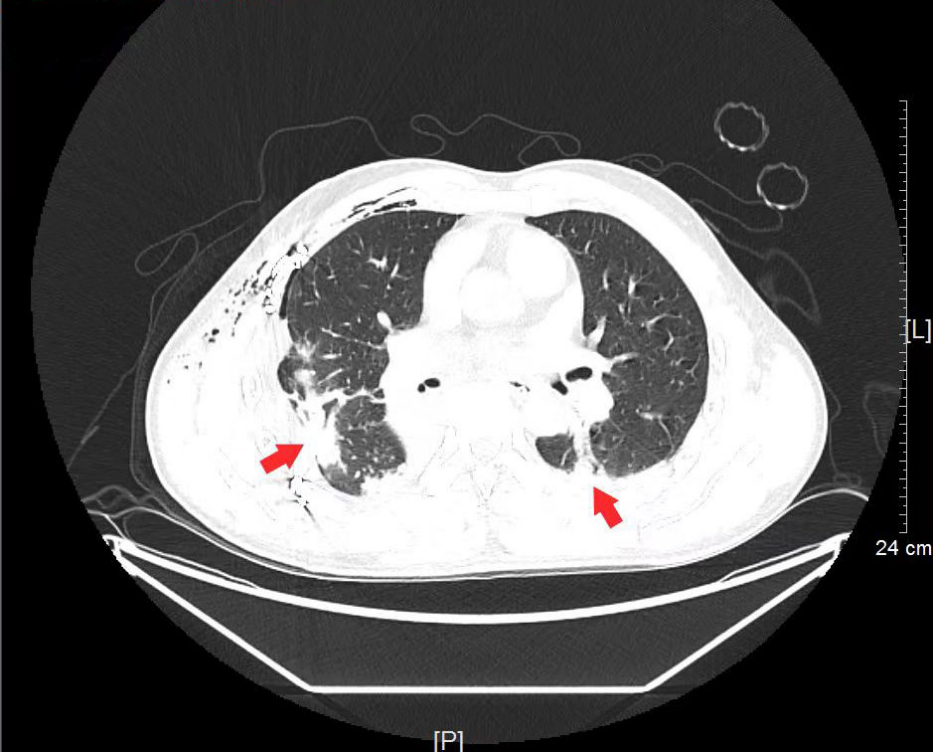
Chest computed tomography (CT) scan of the patient infected with *B. hinzii*.
